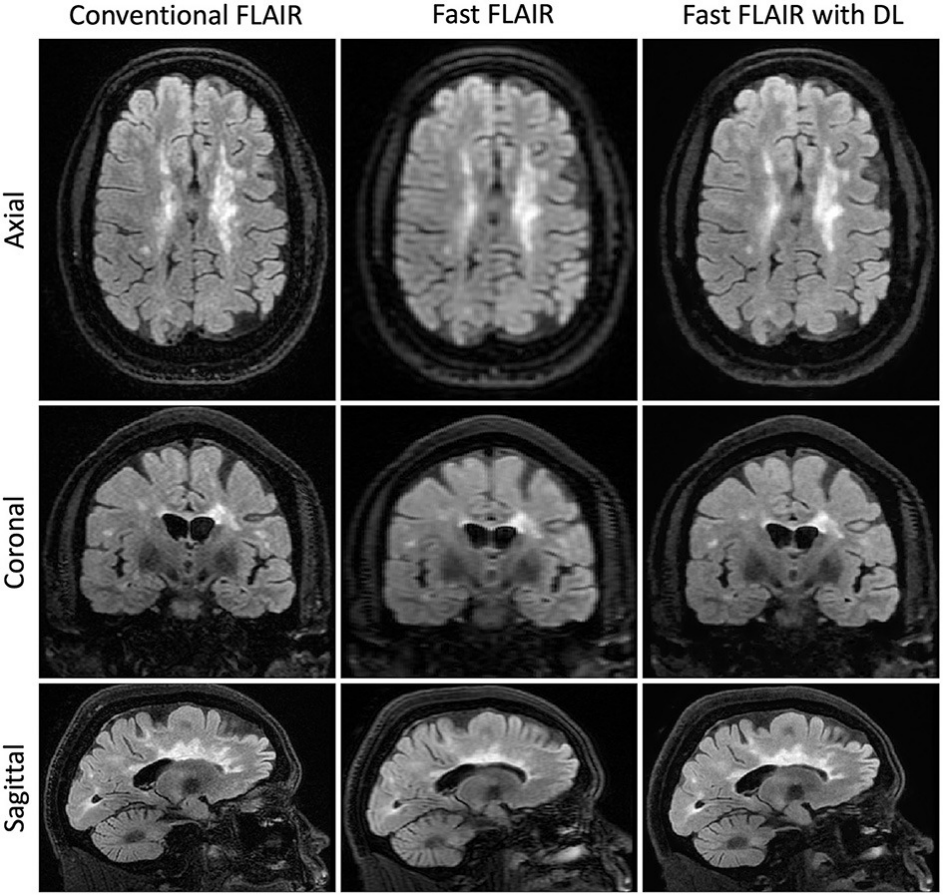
Representative T2FLAIR images. Slices in a similar position for the acquisitions using three different methods: Conventional T2 FLAIR (6:40 min total acquisition time, 256 × 224 × 240 matrix size), fast T2 FLAIR (1:13 min total acquisition time, 256 × 128 × 120 matrix size), and fast T2 FLAIR with DL (same acquisition time and matrix size as the fast T2 FLAIR). FLAIR is the T2-weighted Fluid-Attenuated Inversion Recovery sequence.
